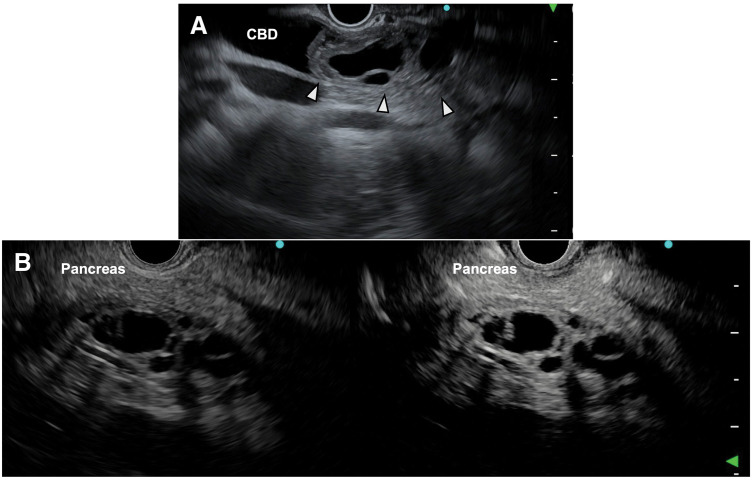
EUS imaging findings. (A) A multilocular cystic tumor covered by a thick capsule is confirmed in the CBD (arrowheads). The tumor arises from the bile duct wall. (B) Contrast-enhanced EUS with perflubutane shows multiple septa enhancing within the cyst, presenting a cyst-in-cyst appearance (left: pre-contrast; right: post-contrast). CBD, common bile duct, EUS, endoscopic ultrasound
